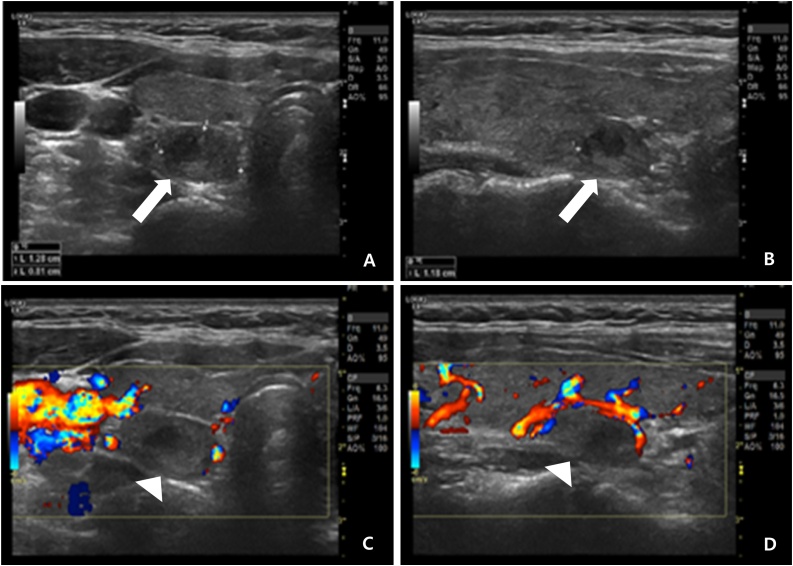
Thyroid ultrasonography: 1.3 × 0.8 × 1.2 cm-sized partially cystic solid nodule (A, B; white arrow) without increasing vascularity (C, D; white arrow head) in the right lobe of the thyroid gland.
